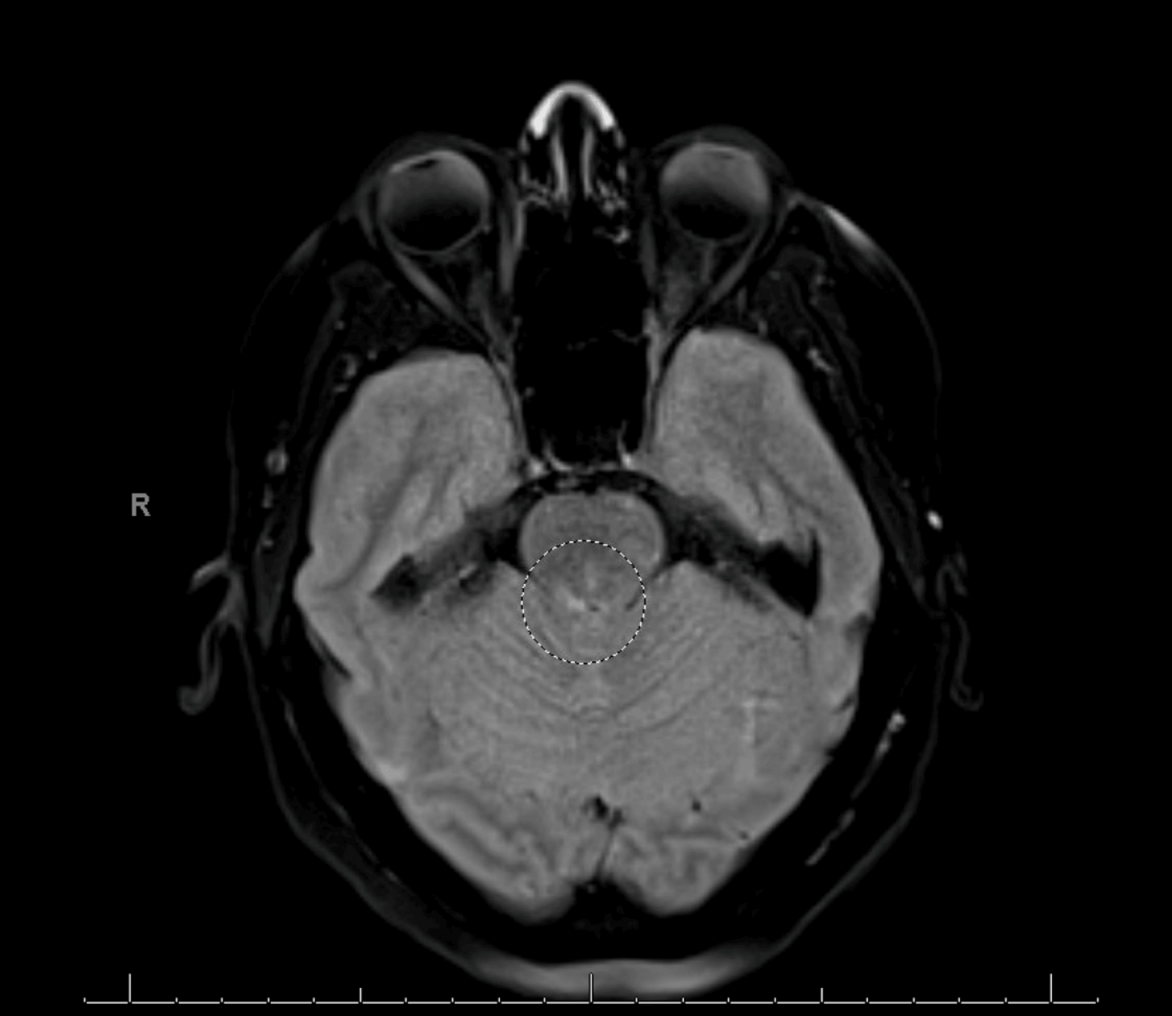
Mild increased FLAIR signal in the bilateral middle cerebellar peduncles without corresponding restricted diffusion seen on MRI brain WWO, showing brain section at the level of the pons FLAIR: fluid-attenuated inversion recovery, WWO: with and without.
